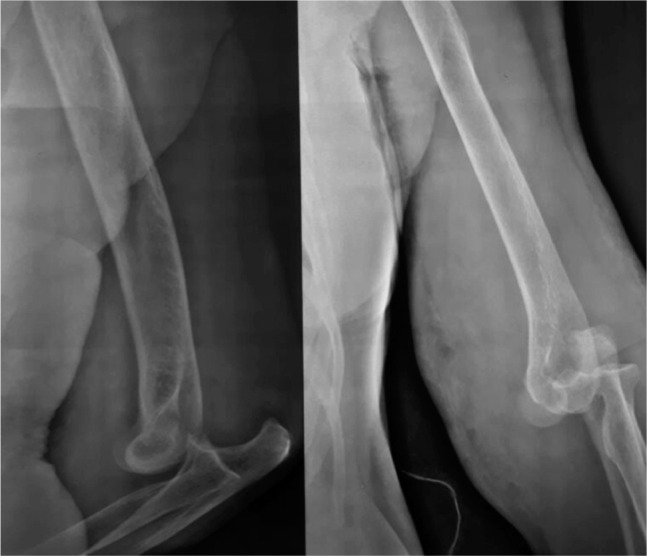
Pre-reduction elbow radiographs (Lateral and AP) showed a posterolateral dislocation of the left elbow. No fractures were noted.
